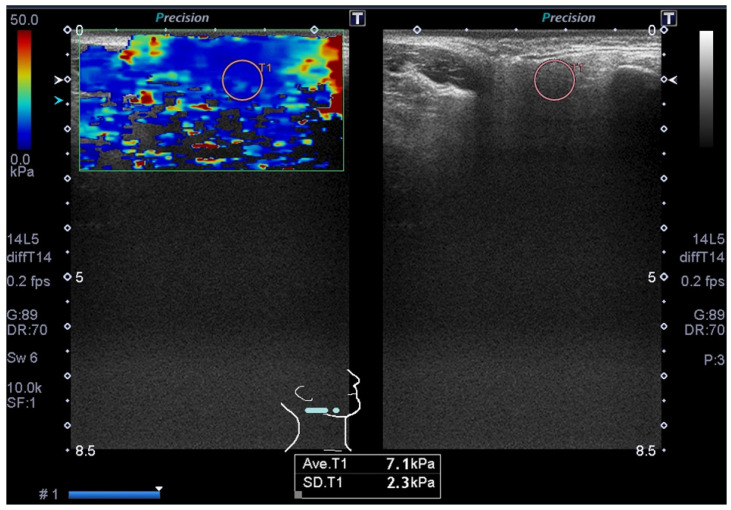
Ultrasonography of right parotid gland in 68-year-old male with tongue carcinoma. Ultrasonography indicates homogeneity in internal architecture of parotid gland on B-mode. Shear wave elastography indicates that shear elastic modulus of parotid gland is 7.1 ± 2.3 kPa.
